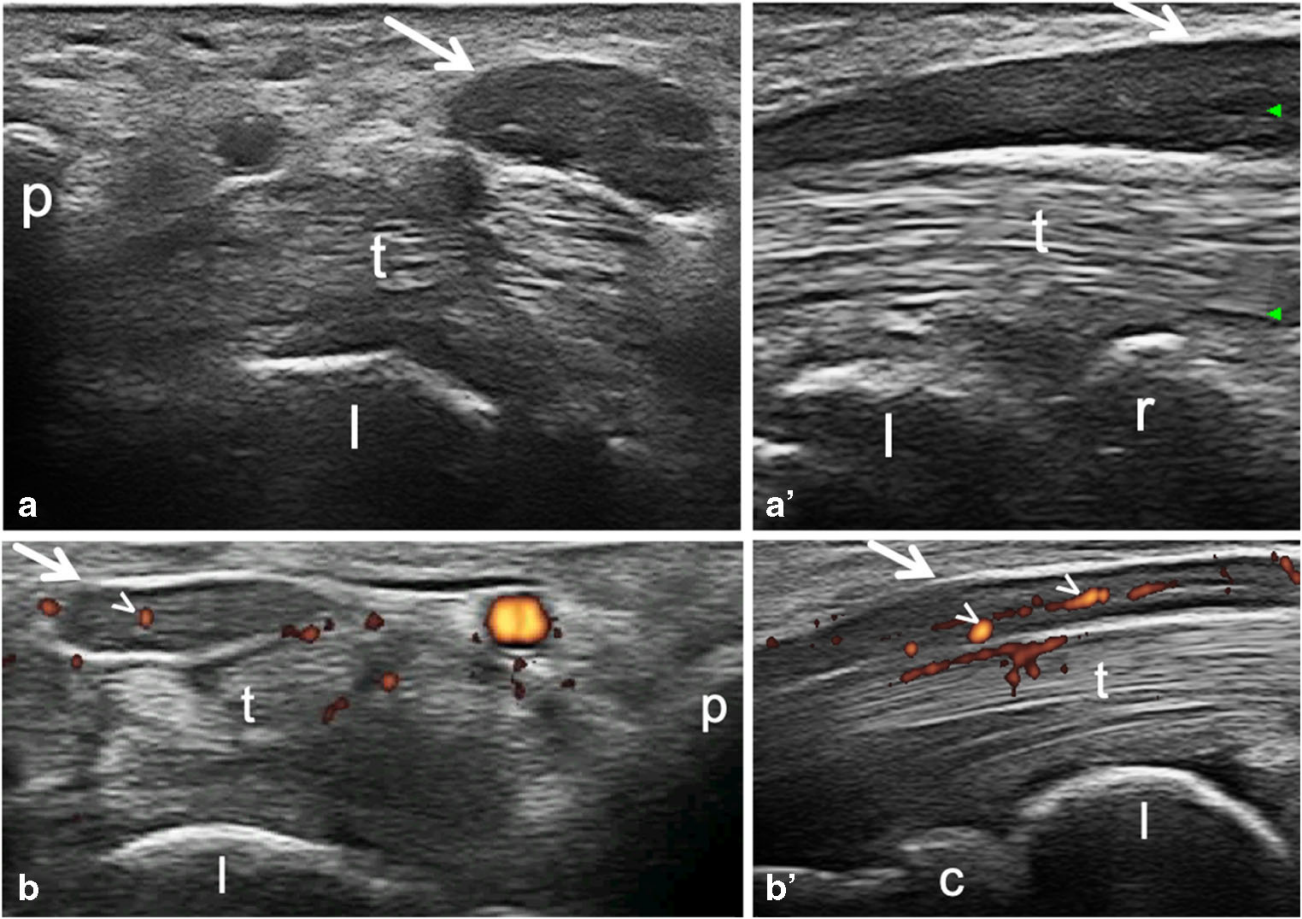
Idiopathic carpal tunnel syndrome. Representative images obtained with transverse (a and b) and longitudinal (a′ and b′) scans. Transverse scans were conducted at the carpal tunnel proximal inlet. (a–a′) B-mode US examination of a 74-year-old female patient showed median nerve marked enlargement (arrows). (b–b′) Power Doppler US examination of a 52-year-old male patient showed abnormal intraneural blood flow (arrowheads), and mild swelling of the median nerve (arrows). c *=* capitate bone, p *=* pisiform bone, r = radius, l = lunate bone, t *=* finger flexor tendons
